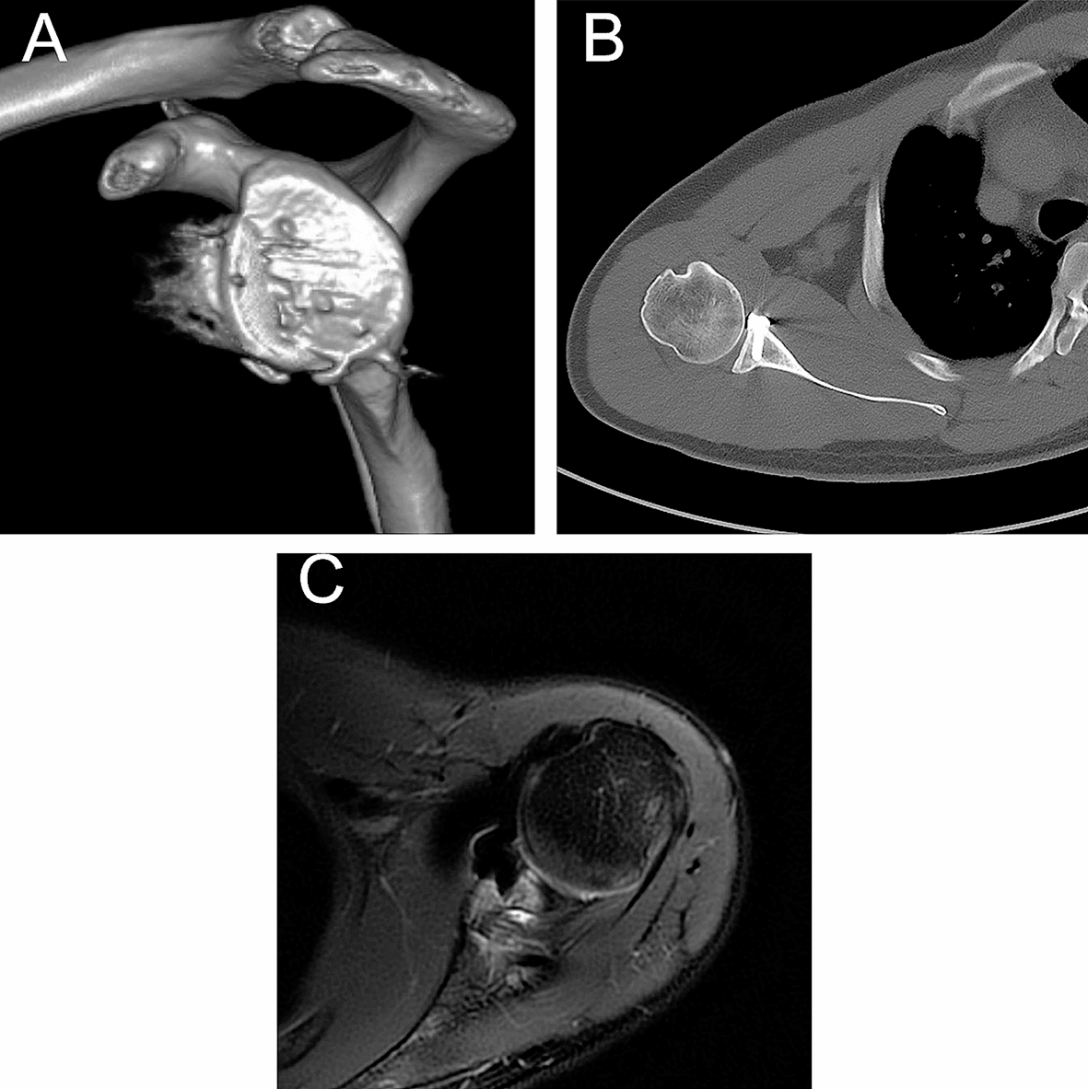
CT and magnetic resonance images of the patient at 2 years postoperatively. (A) CT 3D reconstruction 2 years after surgery showed perfect fit of the pad to the defective portion of the shoulder glenoid without malposition. (B) CT examination 2 years after surgery showed tight bone union around the pad riser, indicating bone ingrowth. (C) Magnetic resonance examination 2 years after surgery showed that the humeral head osteochondral bone was intact without obvious osteochondral damage
